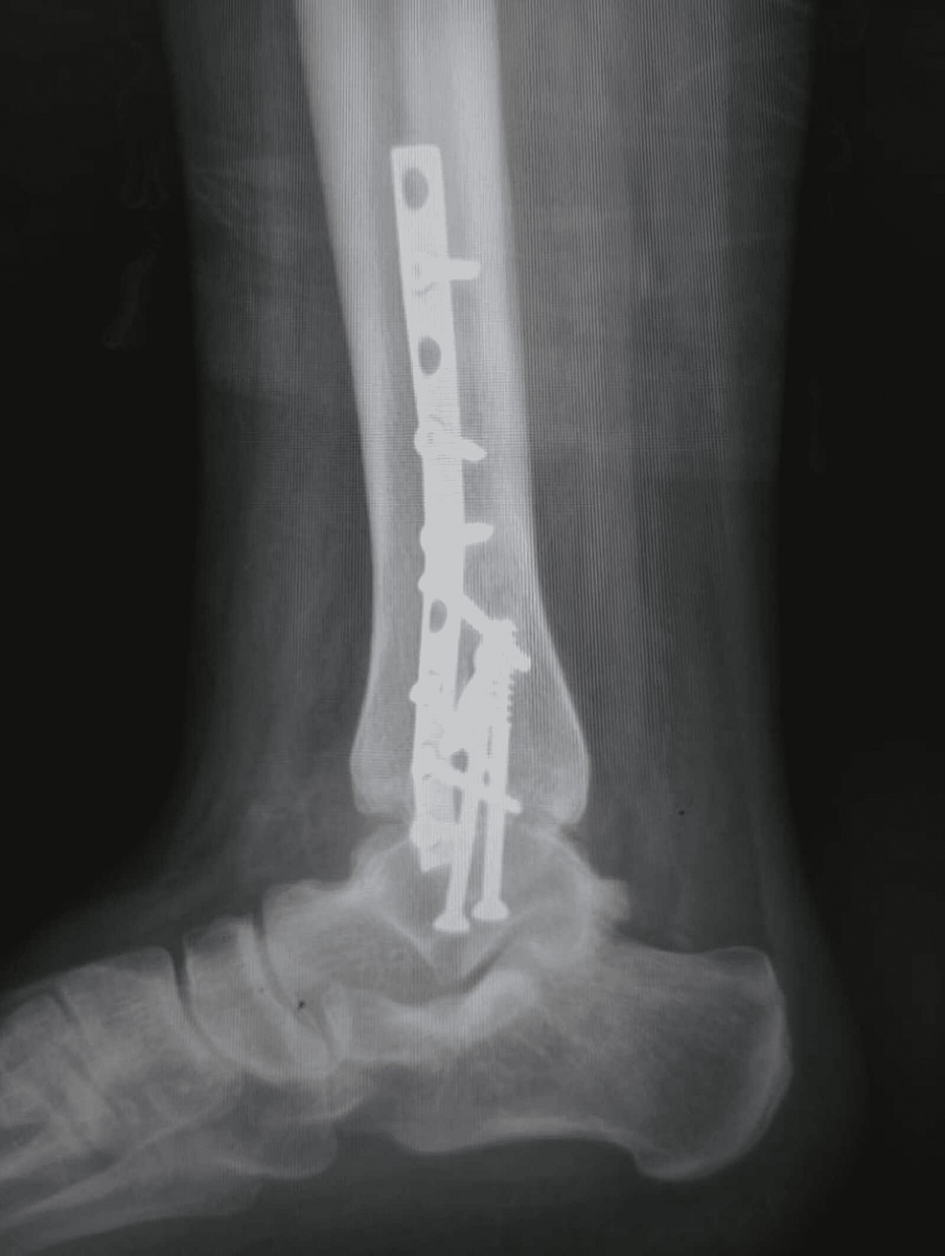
Follow-up radiograph lateral view
